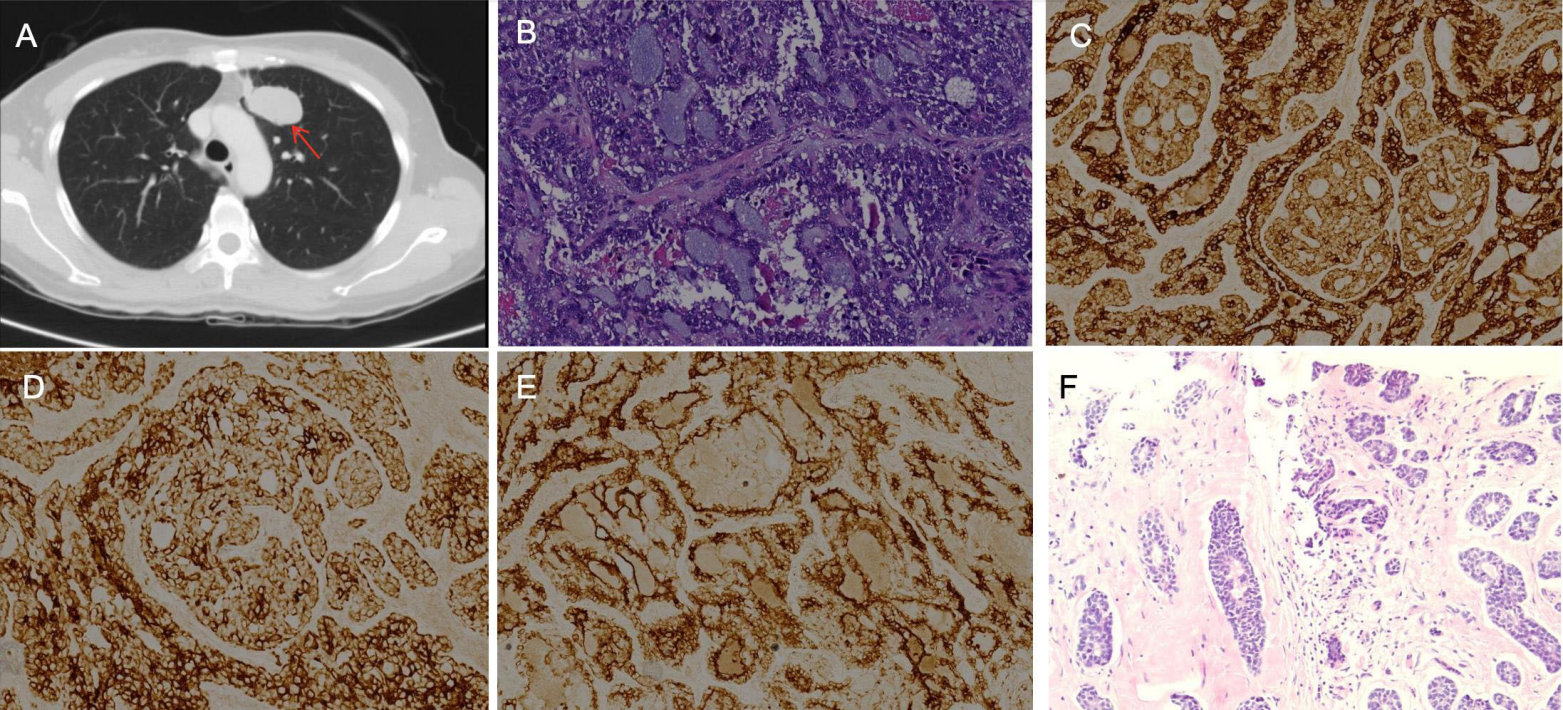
Imaging and pathological images: (A) The lung CT scan shows a peripheral lung cancer in the upper left lung. (B) The postoperative lung tissue pathological image (HE×200) is PACC. IHC×400 shows (C) CK (+), (D) CK7 (+), (E) EMA (+). (F) The puncture of the chest wall mass confirmed metastatic ACC (HE×200).
